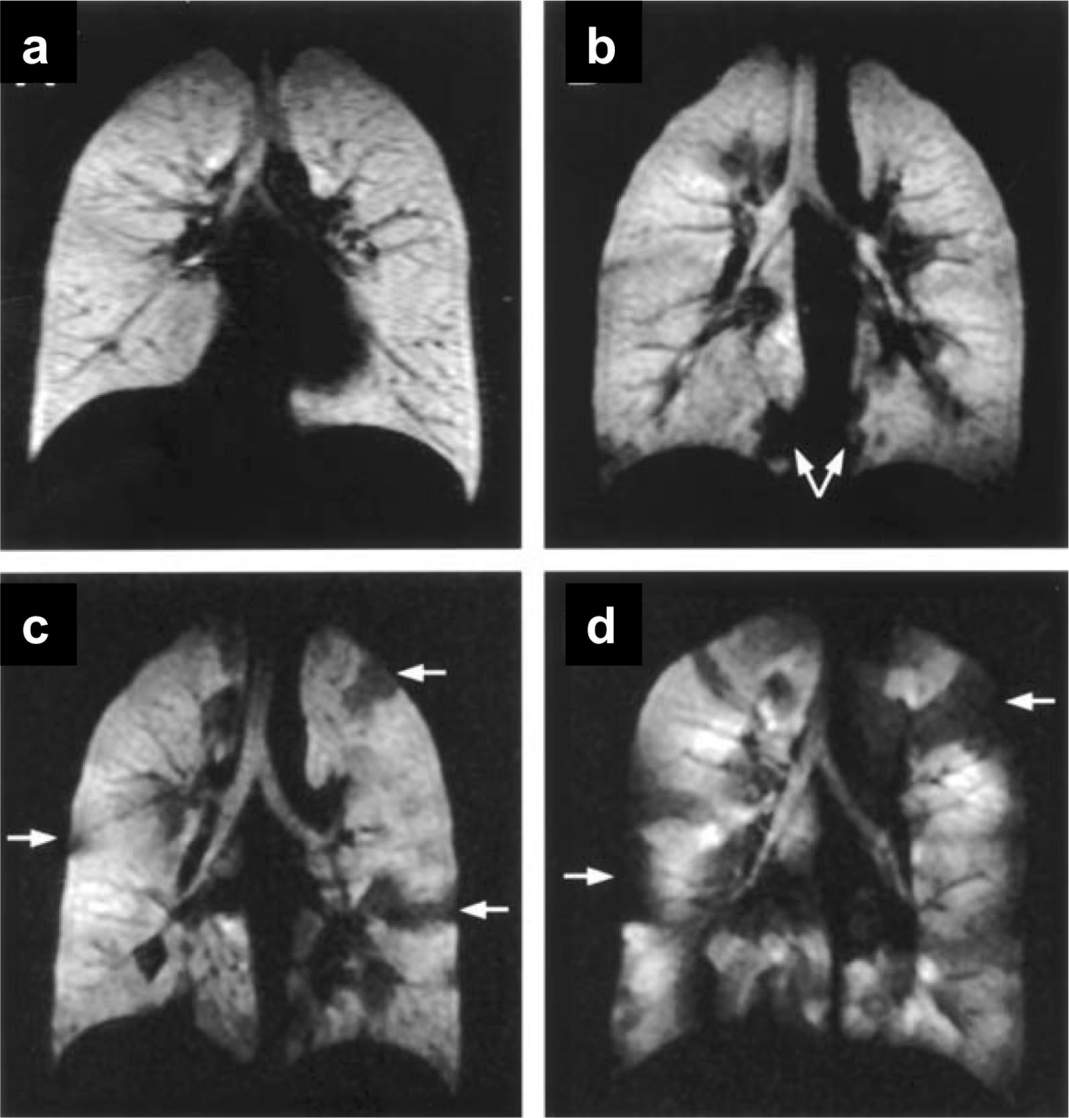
Coronal MR images obtained immediately after inhalation of hyperpolarized Helium-3 gas in a healthy normal volunteer (a) and in patients with mild (FEV1 of 132% of predicted value. b Moderate (FEV1 of 83% of predicted value. c Severe (FEV1 of 34% of predicted value. d Asthma. The distribution of the gas is homogenous in the normal volunteer, and ventilation defects are seen with increasing numbers in the asthmatic patients with increasing severity (arrows pointing at several defects). Reproduced with permission from Elsevier Ltd (License Number: 5324281420540). J Allergy Clin Immunol. 2003 Jun;111(6):1205–11
